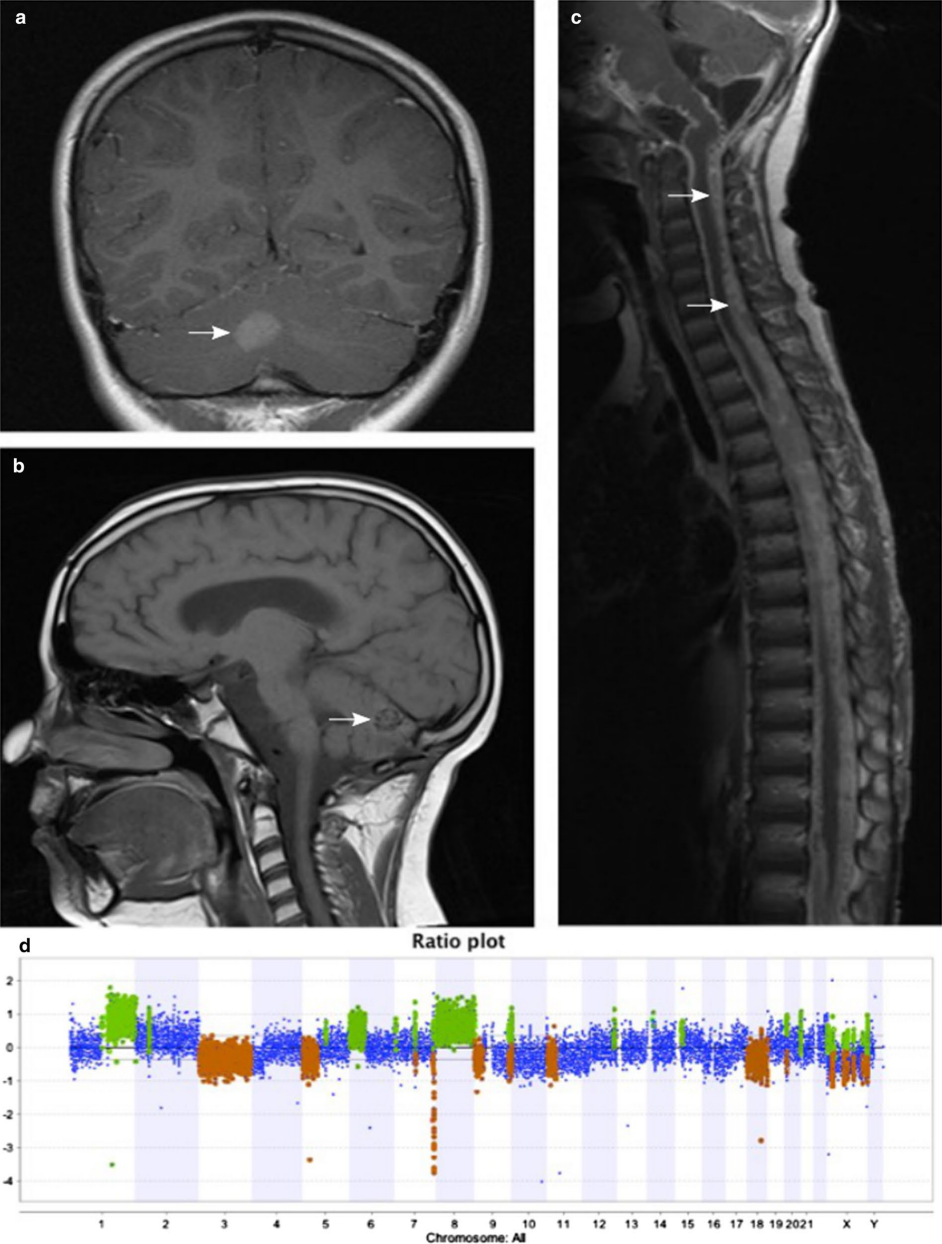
MRI of patient 2 demonstrating melanoma within the cerebellum (a, b) and physically unconnected diffuse leptomeningeal melanoma (c). Cerebellar melanoma showing multiple large gains and losses on array CGH (d)
